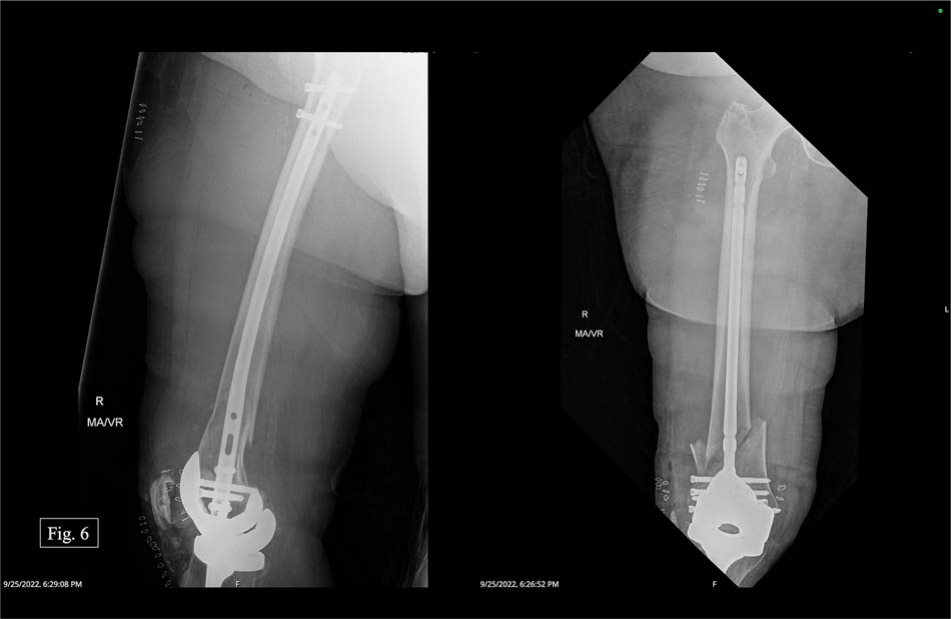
Right, immediate postoperative AP and lateral X-rays.
